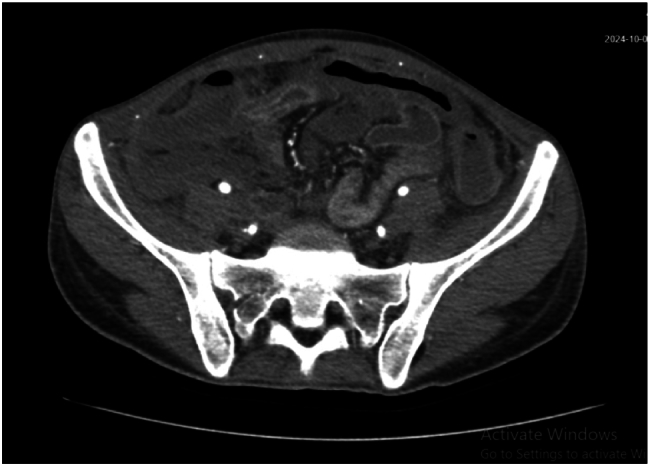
Abdominal CT revealed thickening of the colonic wall, increased density of surrounding mesenteric fat due to inflammation or edema, and mucosal layer enhancement.
